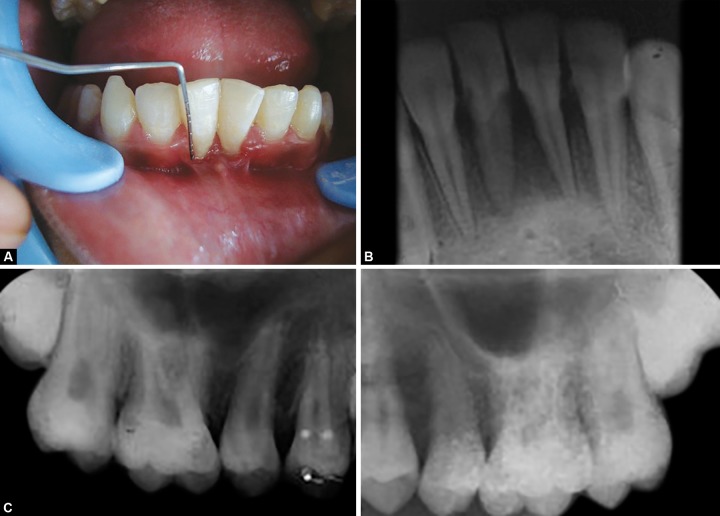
(A) Intraoral photograph showing insufficient sulcus depth and high frenum attachment, (B) intraoral periapical radiograph of 31 and 41 showing mild interdental bone loss and (C) intraoral periapical radiograph of maxillary molars to rule out aggressive periodontitis
